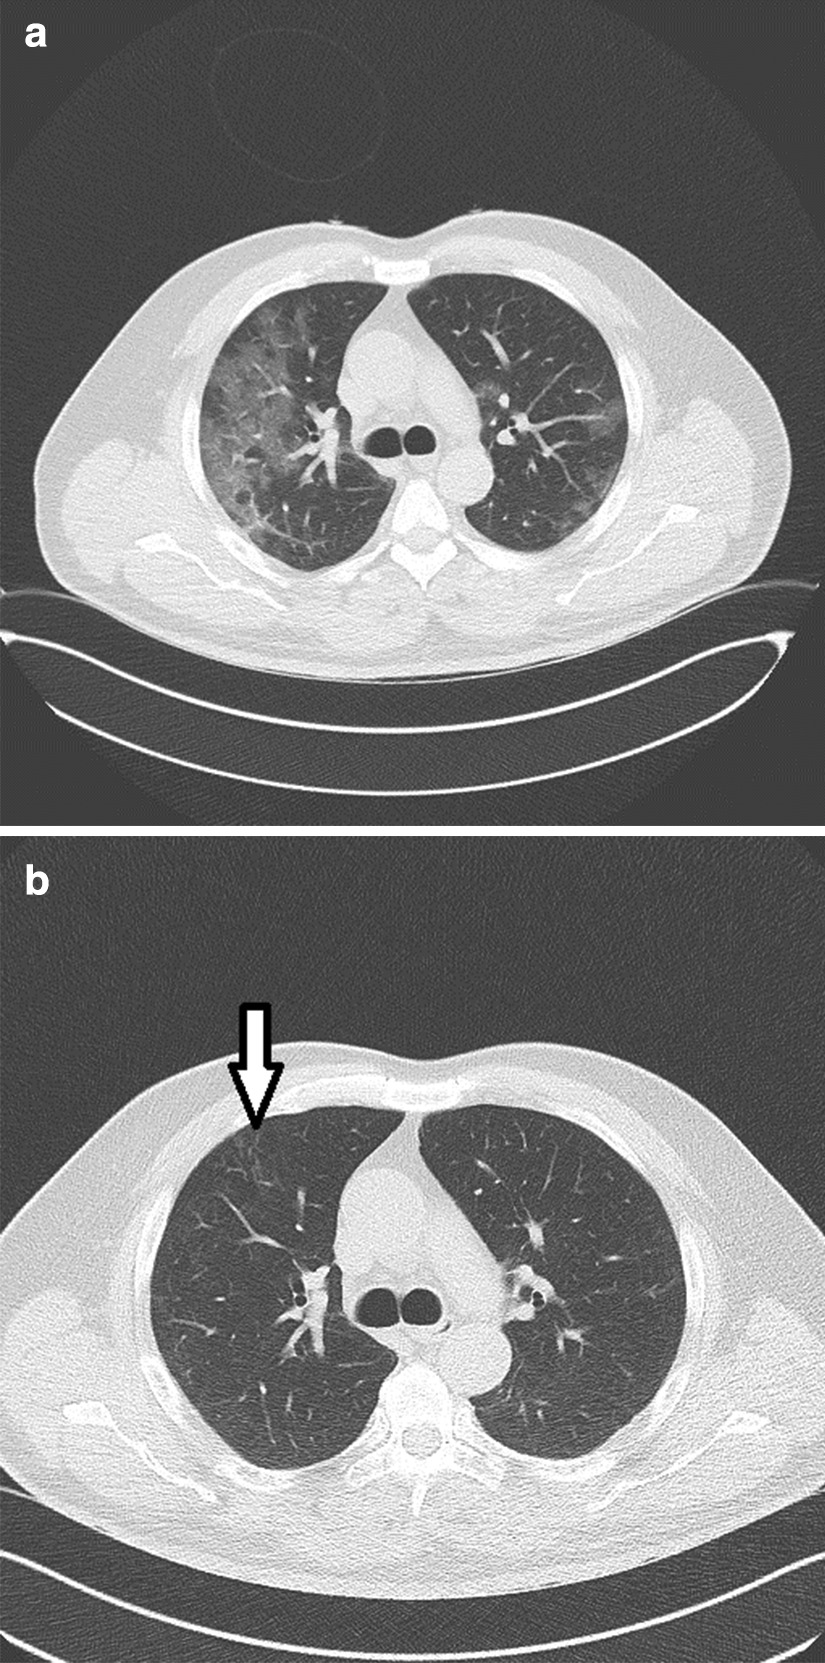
Chest CT Scans of a critical COVID-19 patient, at admission, 14 days after symptoms onset (a) and 3-months post ICU discharge (b). Bilateral ground glass opacities (GGO) in a peripheral distribution are observed in both upper lobes on Pannel A. On Pannel B, GGO has disappeared, replaced by a discrete fibrosis area in the upper right lobe (black arrow)
